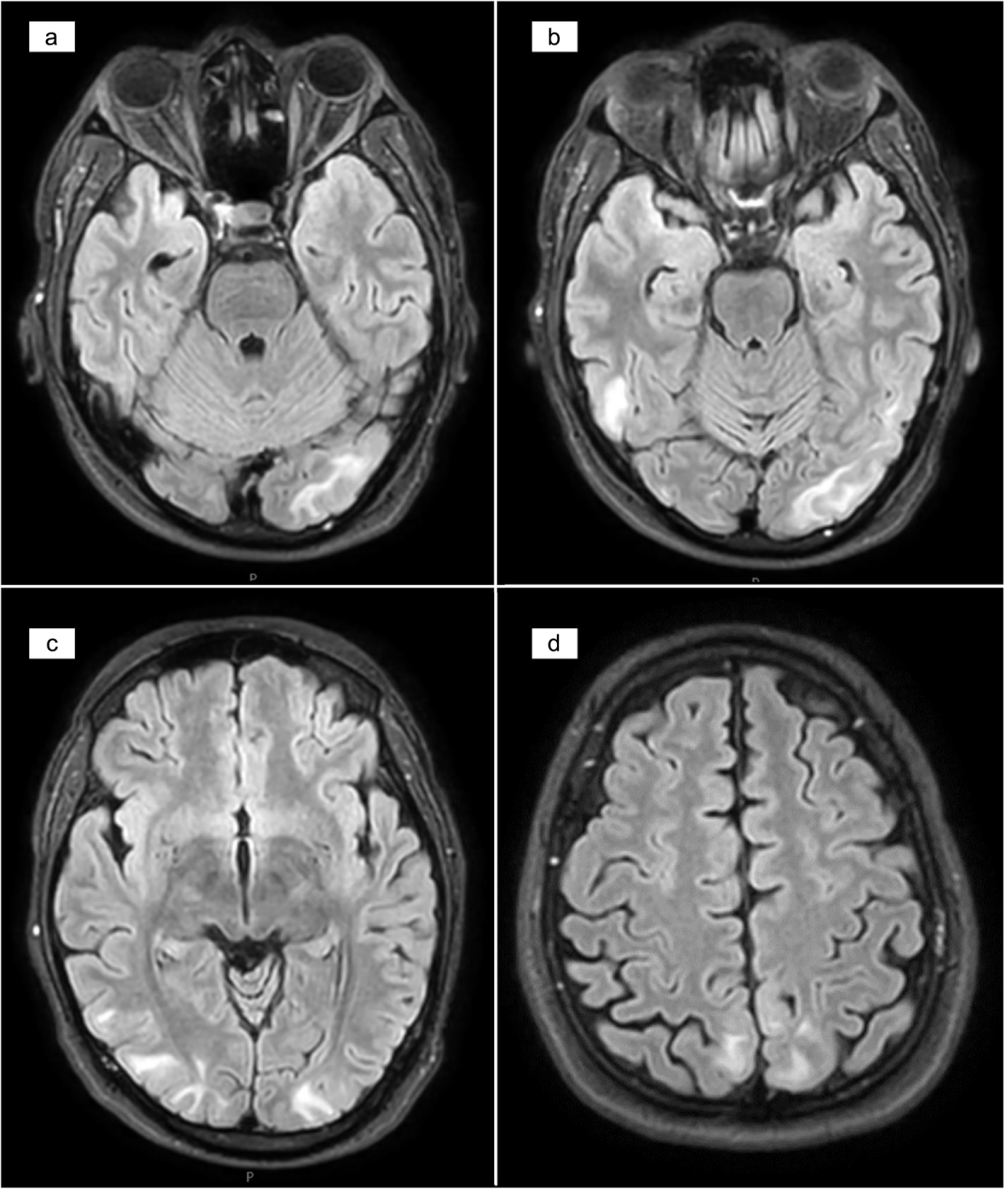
MRI T2 FLAIR images showing high signal intensities involving mainly the subcortical white matter. a) Left occipital area. b) Right temporal area. c) Bilateral occipito-parietal area. d) Bilateral parietal area
